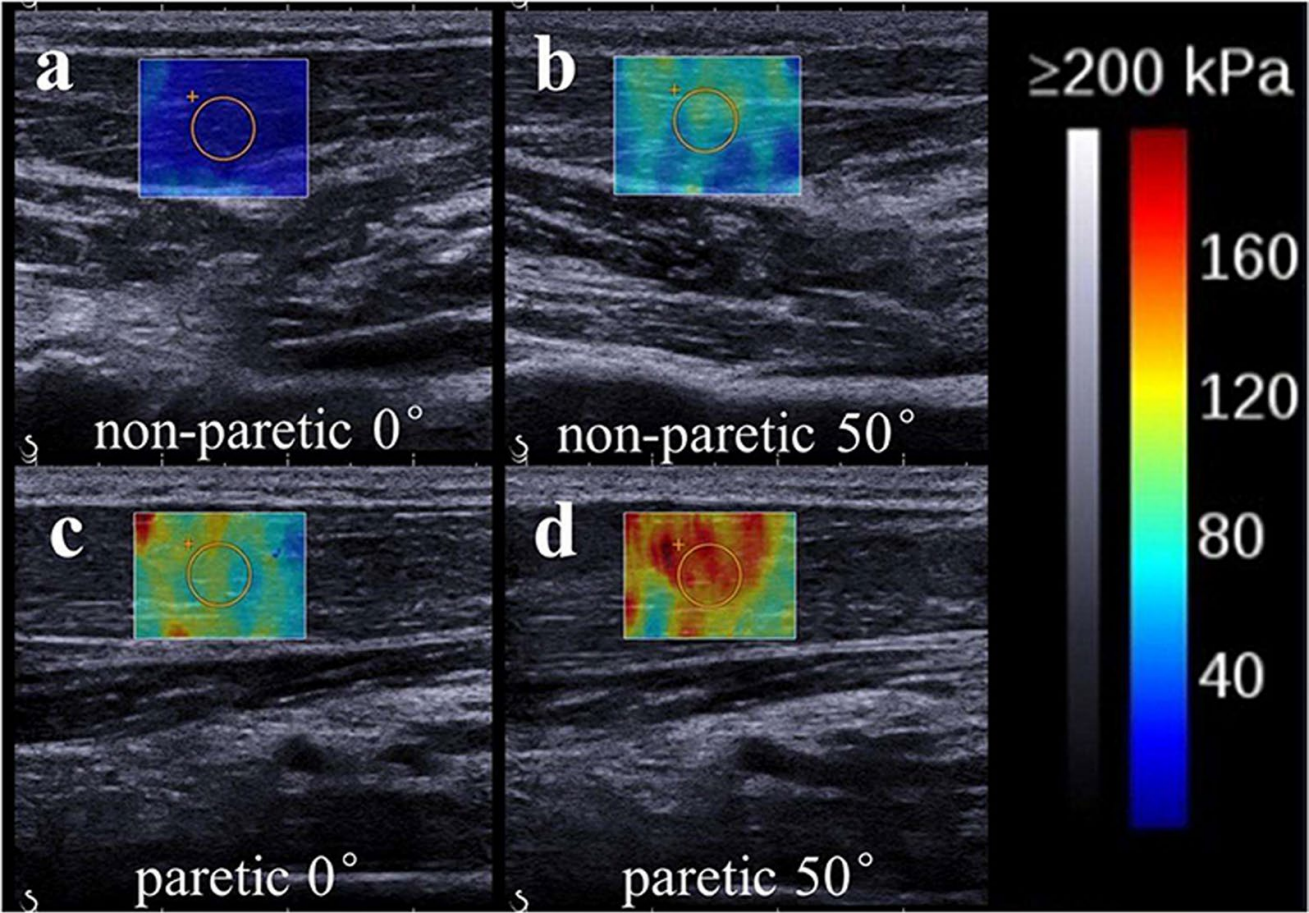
SWE superimposed on the B-mode ultrasound image of the muscles of the (a) neutral non-paretic, (b) stretched non-paretic, (c) neutral paretic and (d) stretched paretic forearm. From Leng et al. (2019) [36]
